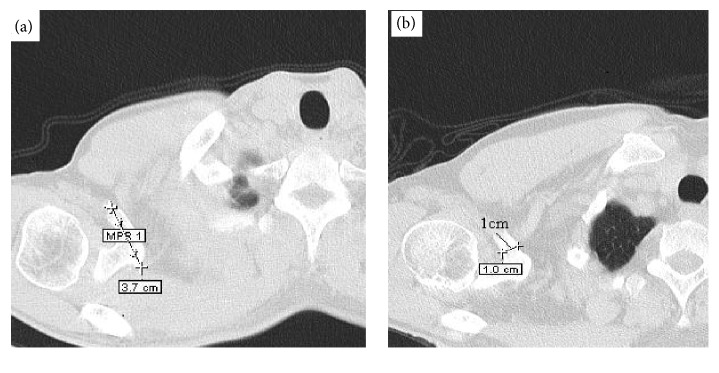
Axial CT scan images showing the measurement of the tip of the coracoid process.
